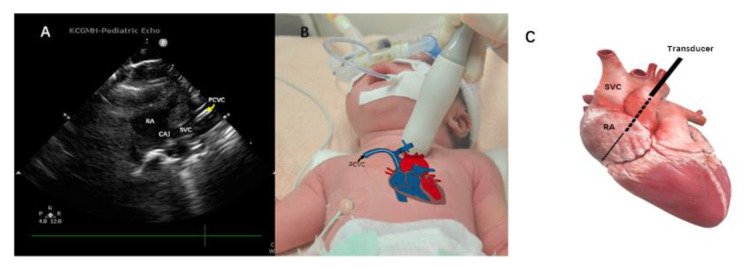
After detecting the aorta, the probe was slightly tilted to the baby’s right side showing (A) PCVC, an image of contrasting double-contoured echo structure (yellow curved arrow) in the SVC; (B) the location of the transducer; and (C) the heart anatomy with the probe position indicated in a black solid line and a black dash line indicating the plane of ultrasound. The black arrow shows PCVC in situ. CAJ, cavoatrial junction; PCVC, percutaneous central venous catheter; RA, right atrium; SVC, superior vena cava.
